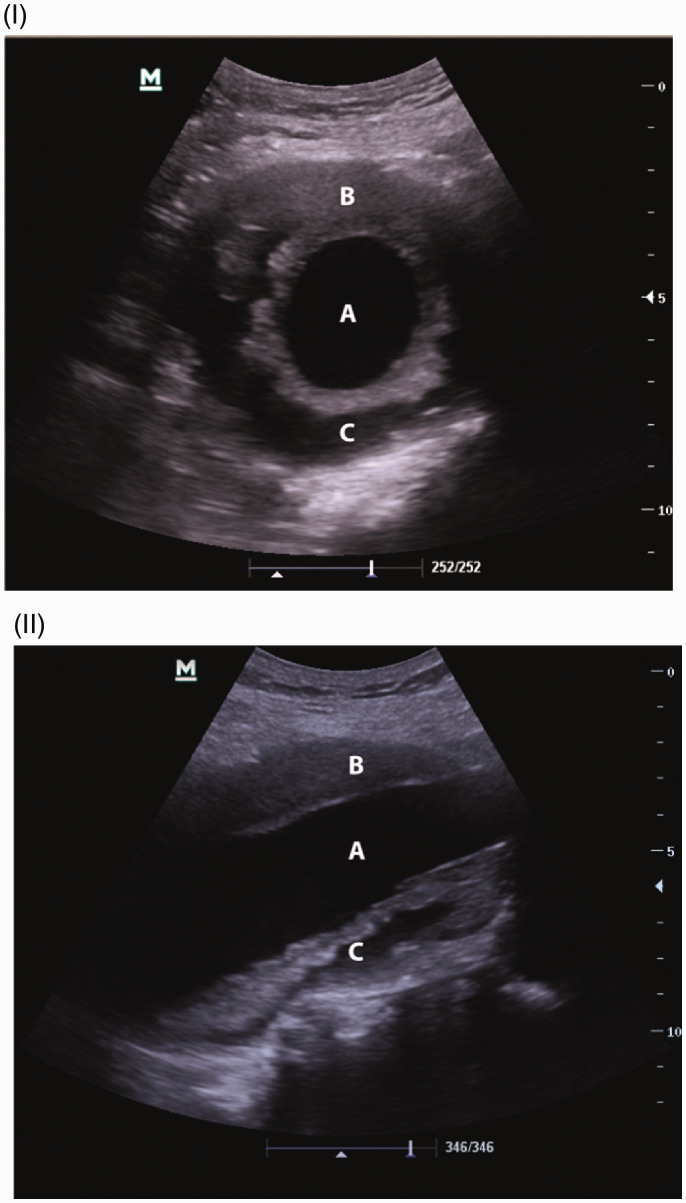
Appearance on ultrasound. (I) Transverse view of an AAA. (II) Longitudinal view. In both, a central lumen (a), an area of stable thrombus (b), and areas of dissection or haemorrhage within the thrombus (c) can be seen. Reproduced with permission from Goldstein and Wells (2019).
